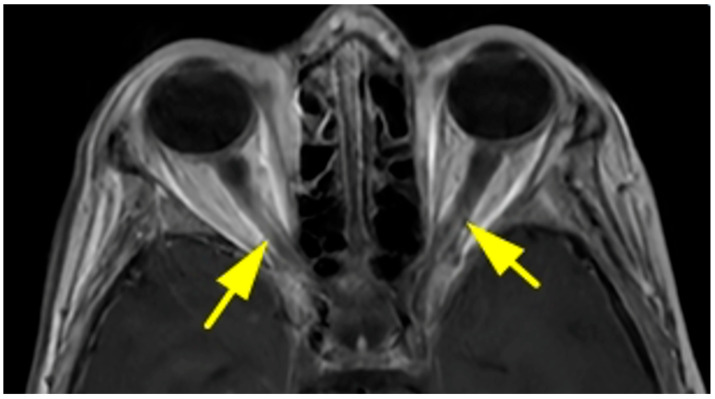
Axial T1-weighted magnetic resonance section showing bilateral optic nerve involvement in the case of a 48-year-old woman with bilateral orbital metastasis of breast cancer (yellow arrows).
